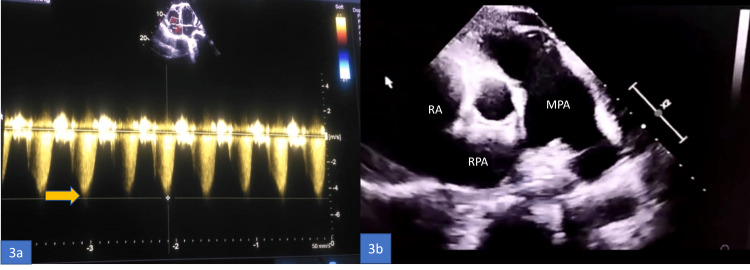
3a: Color doppler echocardiography showing severe tricuspid regurgitation (TR), tricuspid velocity jet of 4.3m/s (yellow arrow), secondary to severe pulmonary arterial hypertension. 3b: Echocardiography parasternal short-axis view–pulmonary artery bifurcation view (PSAX - PABV) showing dilated main pulmonary artery and its branches
